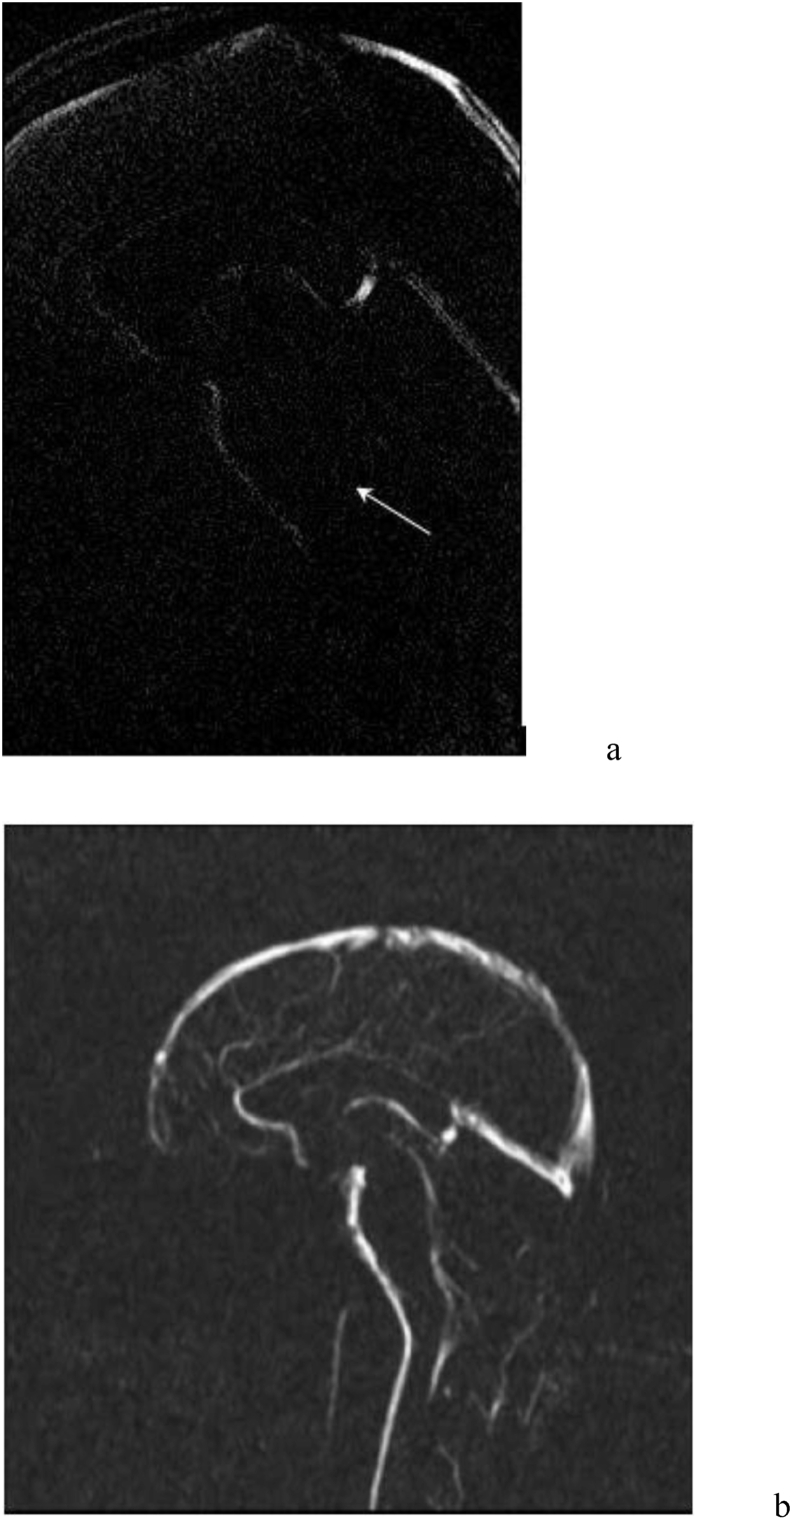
Selected image from an MRI of the brain including velocity encoded phase contrast flow study sequence (a). This reveals tonsillar descent of 17 ​mm. The absence of CSF flow posterior to the cervico-medullary junction is marked by the arrow (a). A normal appearing CSF flow sequence of comparison (b) (Battal et al., 2011).
